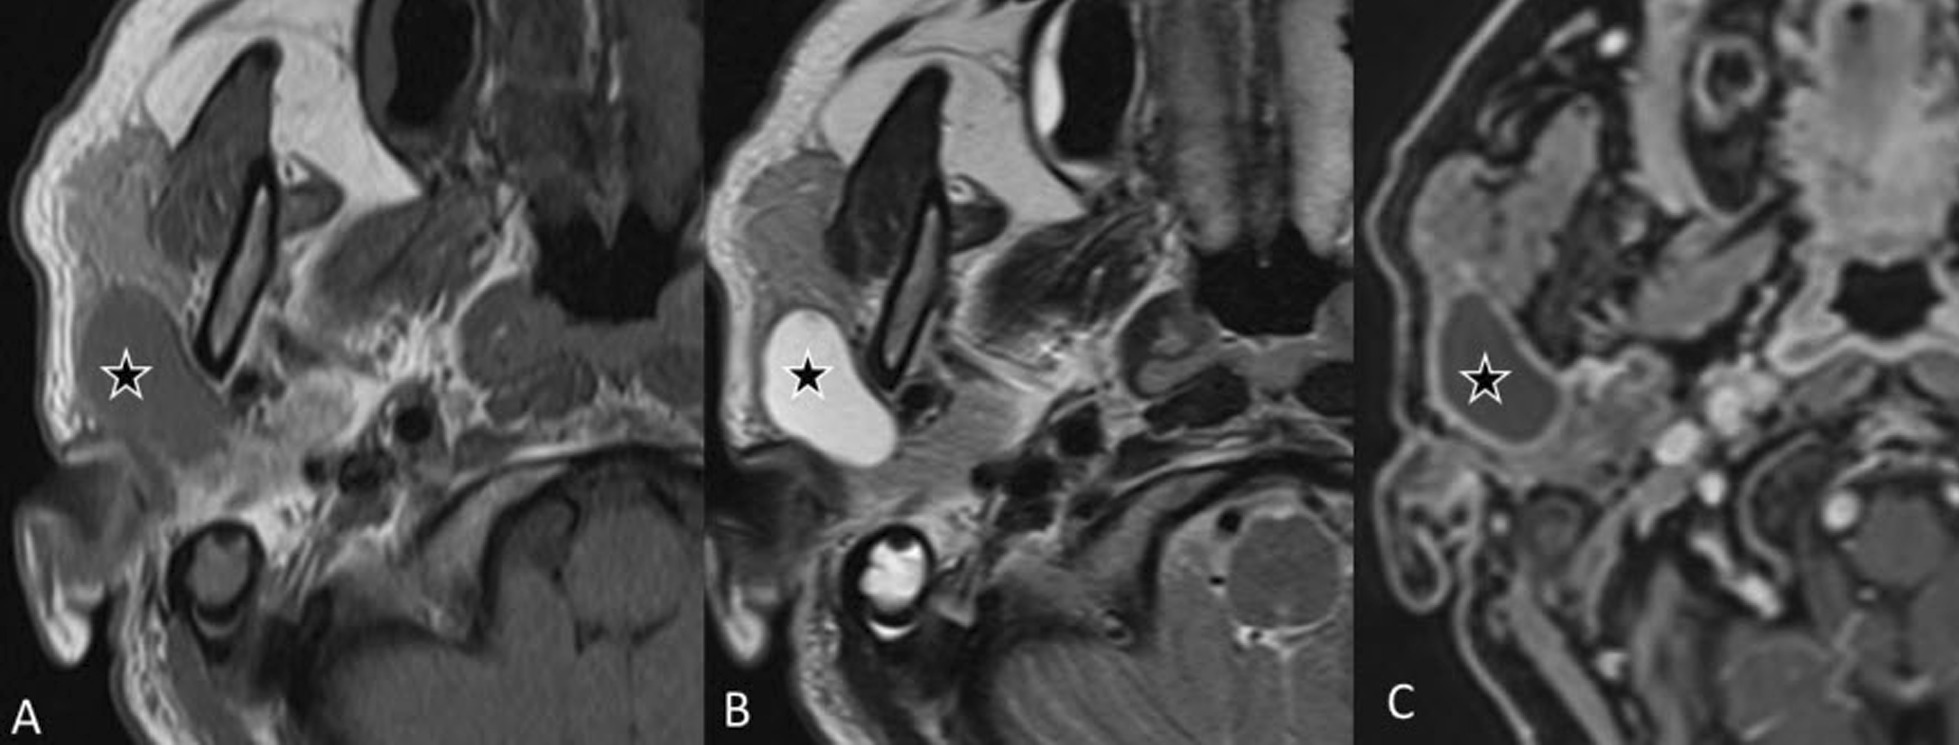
Sporadic/simple lymphoepithelial cyst. A 63-year-old female with painless swelling in the right cheek. Axial T1‑w (A) and axial T2‑w (B) MRI demonstrate a well‑circumscribed unilocular cystic lesion in the superficial lobe of the right parotid gland (star). Axial T1‑w postcontrast fat‑suppressed MRI (C) shows a smooth, thin rim enhancement of the lesion
